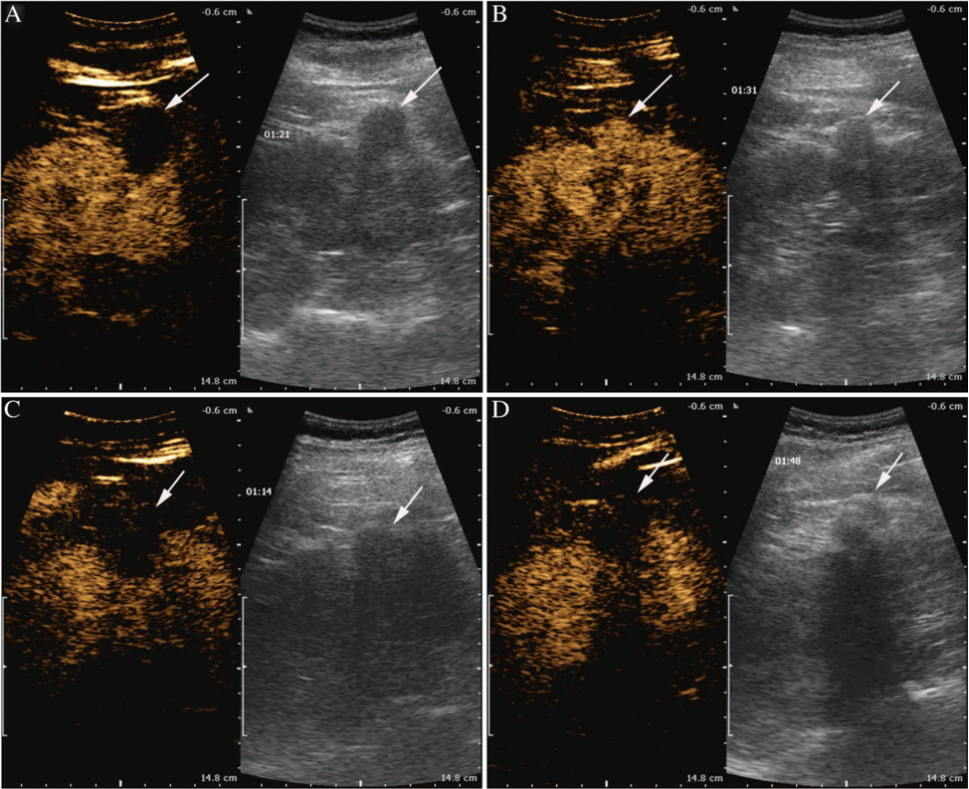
Sequential contrast-enhanced ultrasound images of the ablation area of renal cell carcinoma (RCC) observed for the period with two cryoablations. a Contrast-enhanced ultrasound 1 month after stage 1 cryotherapy. b Contrast-enhanced ultrasound 12 months after stage 1 cryotherapy. c Contrast-enhanced ultrasound 1 month after stage 2 cryotherapy. d Contrast-enhanced ultrasound 12 months after stage 2 cryotherapy
